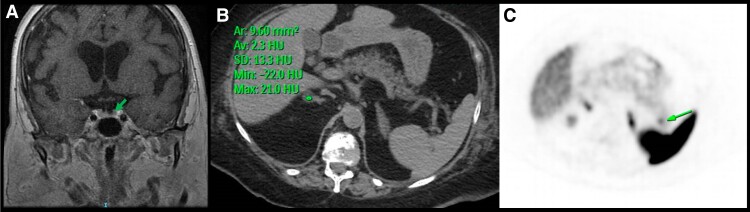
A, Pituitary MRI: Possible 0.5-cm lesion and acute/subacute CVAs. B, Chest/abdominal/pelvis CT: 1.5-cm lipid-rich adrenal adenoma. C, ^68^Ga-DOTATATE PET-CT scan: pancreatic tail 7-mm DOTATATE-avid lesion, suspicious for NET.
